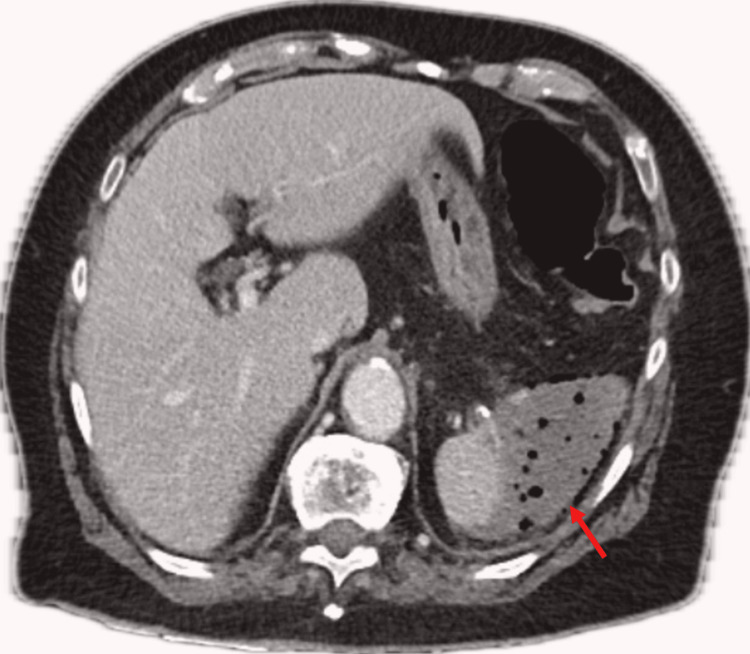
Emphysematous spleen on CT imaging at presentation (red arrow)
